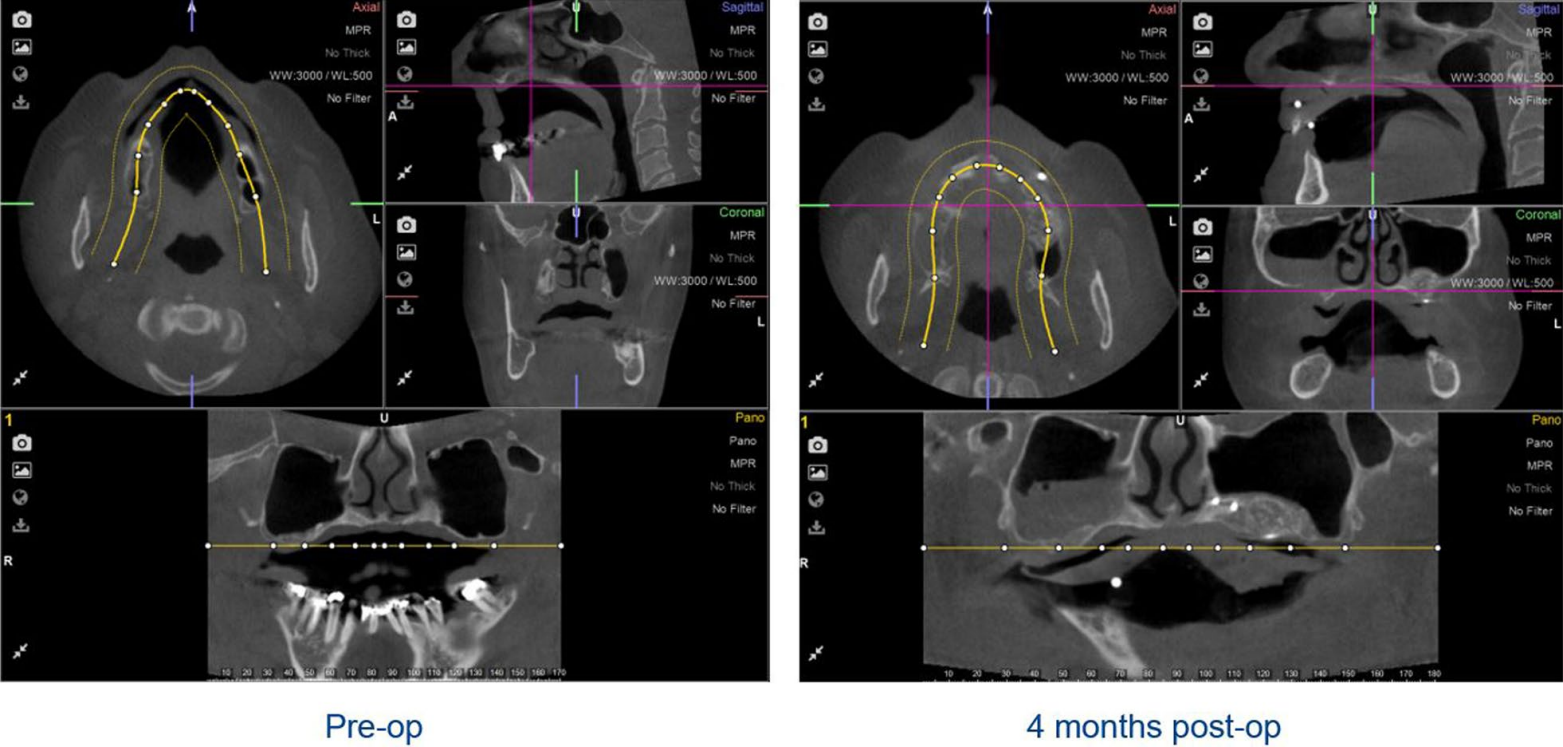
Planes of the preoperative and 4-month postoperative CBCTs in the right orientation: transversal, sagittal and frontal. The lower parts show the defined panoramic plane in which the measurements of bone height were made
